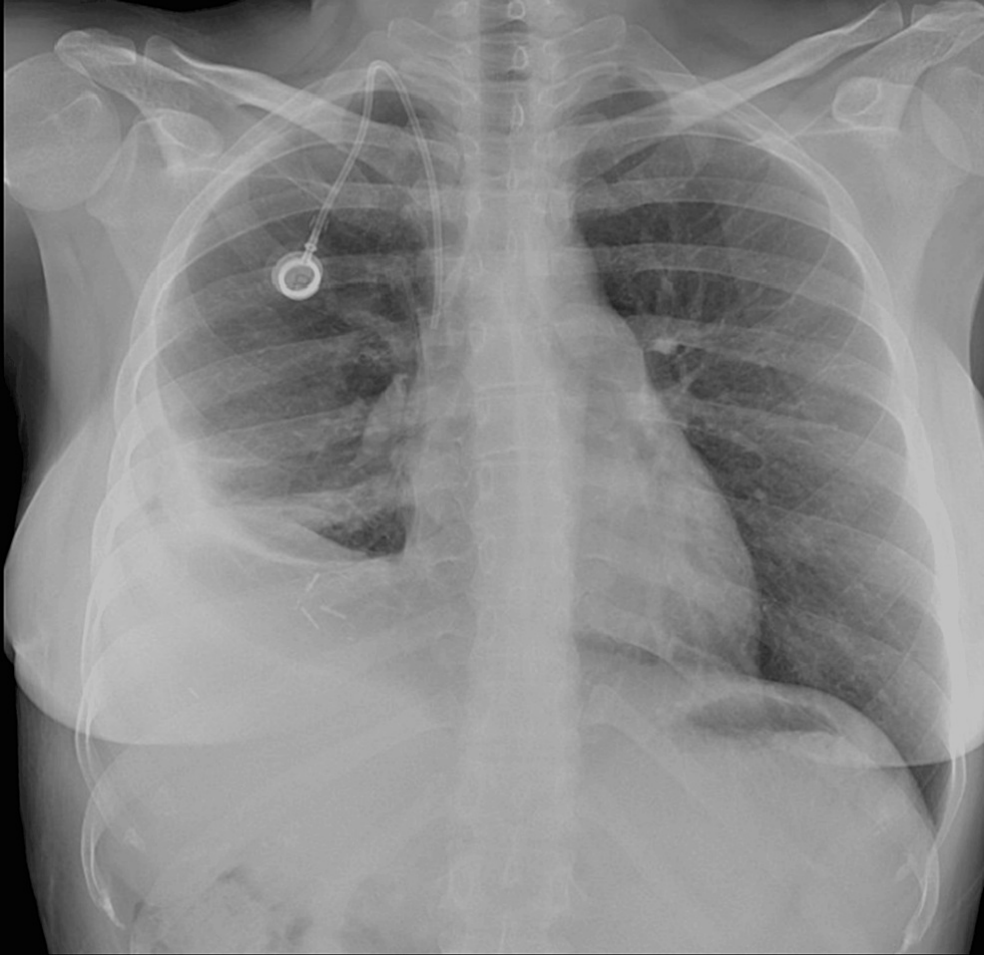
Post-operative chest X-ray showing successful resolution
